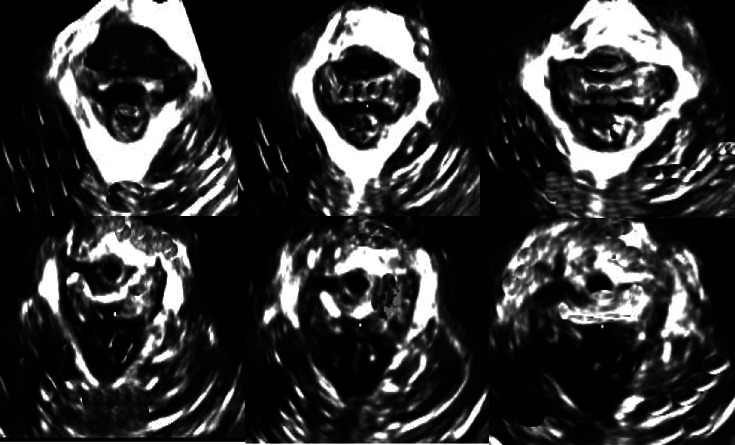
The 3D ultrasound image of the patient with defected AS.
